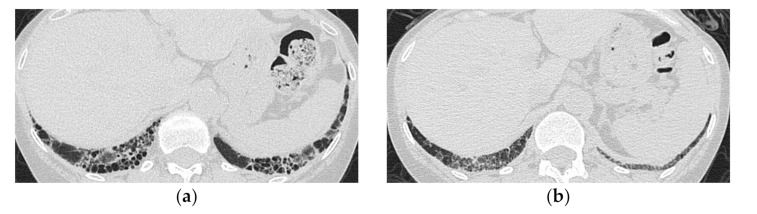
A 59-year-old woman with dermatomyositis under long-term follow-up. The most recent high-resolution computed tomography (HRCT) (a) shows bilateral honeycomb-like cystic lesion just below the pleura, and a review of the HRCT scan at the time of initial presentation 17 years ago (b) shows that the same area was previously ground-glass opacification with mild reticulation showing NSIP pattern.
